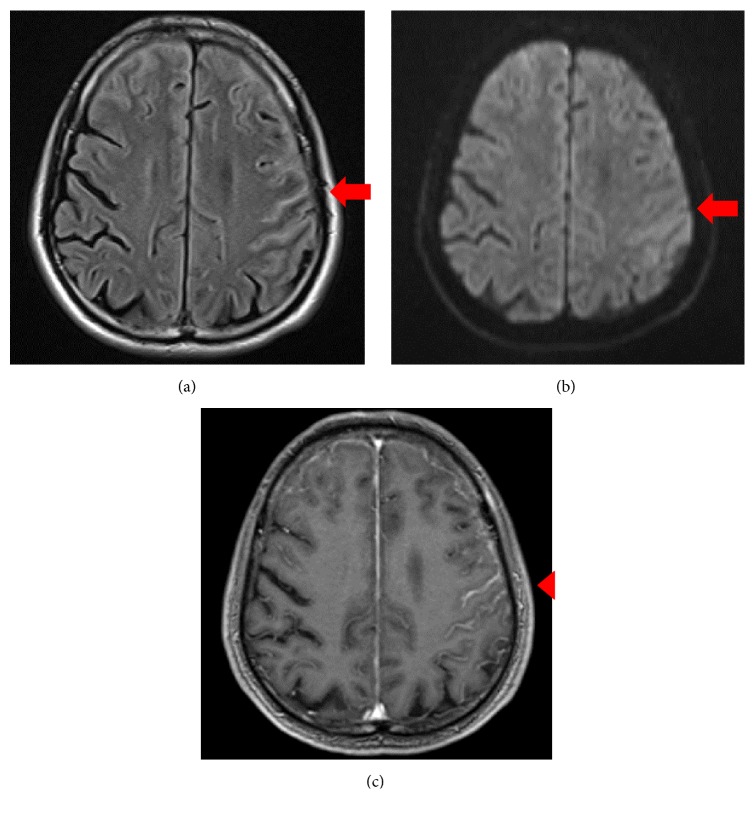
Brain MRI findings on the 4th admission. (a) Axial T2 fluid-attenuated inversion recovery (FLAIR) image (1.5 T; TR 10000 ms, TE 108 ms). (b) Diffusion-weighted image (DWI) (1.5 T; TR 5000 ms, TE 75 ms). (c) T1-weighted image (T1WI) enhanced by gadolinium (1.5 T; TR 7.50 ms, TE 2.82 ms). MRI shows extensive thick linear hyperintensity of the leptomeninges in bilateral frontal lobe and the left parietal lobe on T2 FLAIR and DWI (red arrows). T1WI after gadolinium administration shows enhancement of the leptomeninges (red arrowhead).
